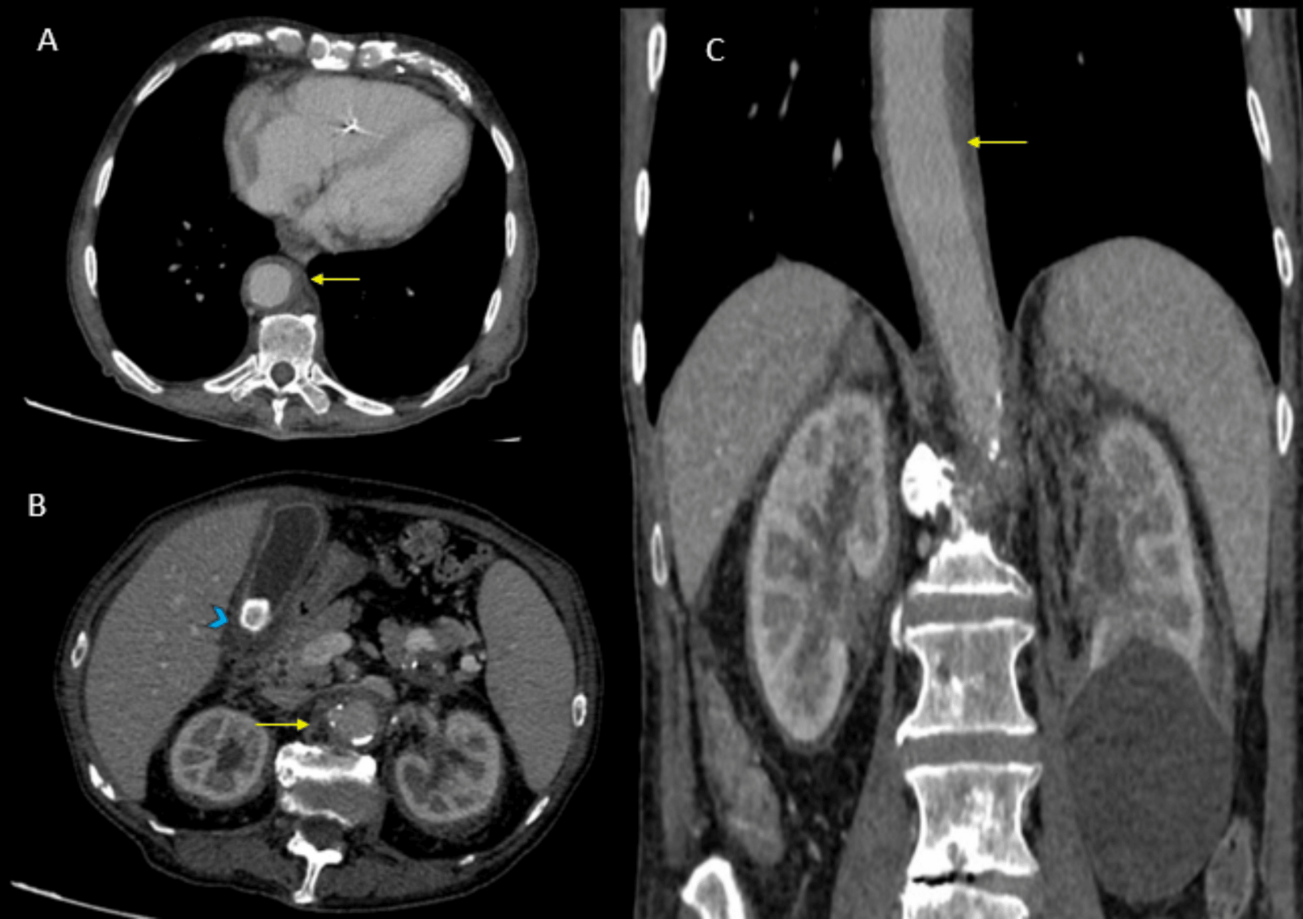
Axial CT of the lower thorax (A) and abdomen (B) shows circumferential coating of the aorta that does not spare the posterior wall (yellow arrows). Coronal reformation (C) also depicts the extent of the tissue infiltration around the aorta (yellow arrow). Incidental gallbladder stones are also seen in B (blue arrowhead) CT: computed tomography
